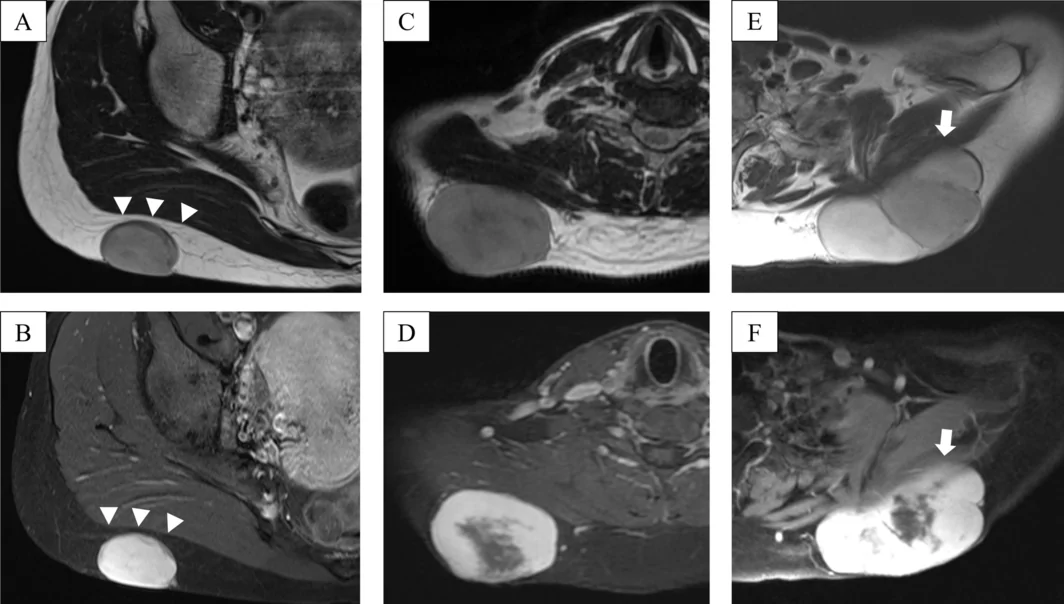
Assessment of the relationship between the tumor and the fascia on preoperative MRI. (1) Not contacting fascia (gluteal lesion). On T2‐weighted imaging (A) and contrast‐enhanced fat‐suppressed T1‐weighted imaging (B), interposed fat tissue is observed between the tumor and the muscle (arrowheads). (2) Contacting fascia (back lesion). T2‐weighted imaging (C) and contrast‐enhanced fat‐suppressed T1‐weighted imaging (D) show no interposed fat tissue between the tumor and the muscle. (3) Beyond fascia (back lesion). On T2‐weighted imaging (E) and contrast‐enhanced fat‐suppressed T1‐weighted imaging (F), continuous signal change extending into the muscle is observed, suggesting intramuscular invasion (arrow).
